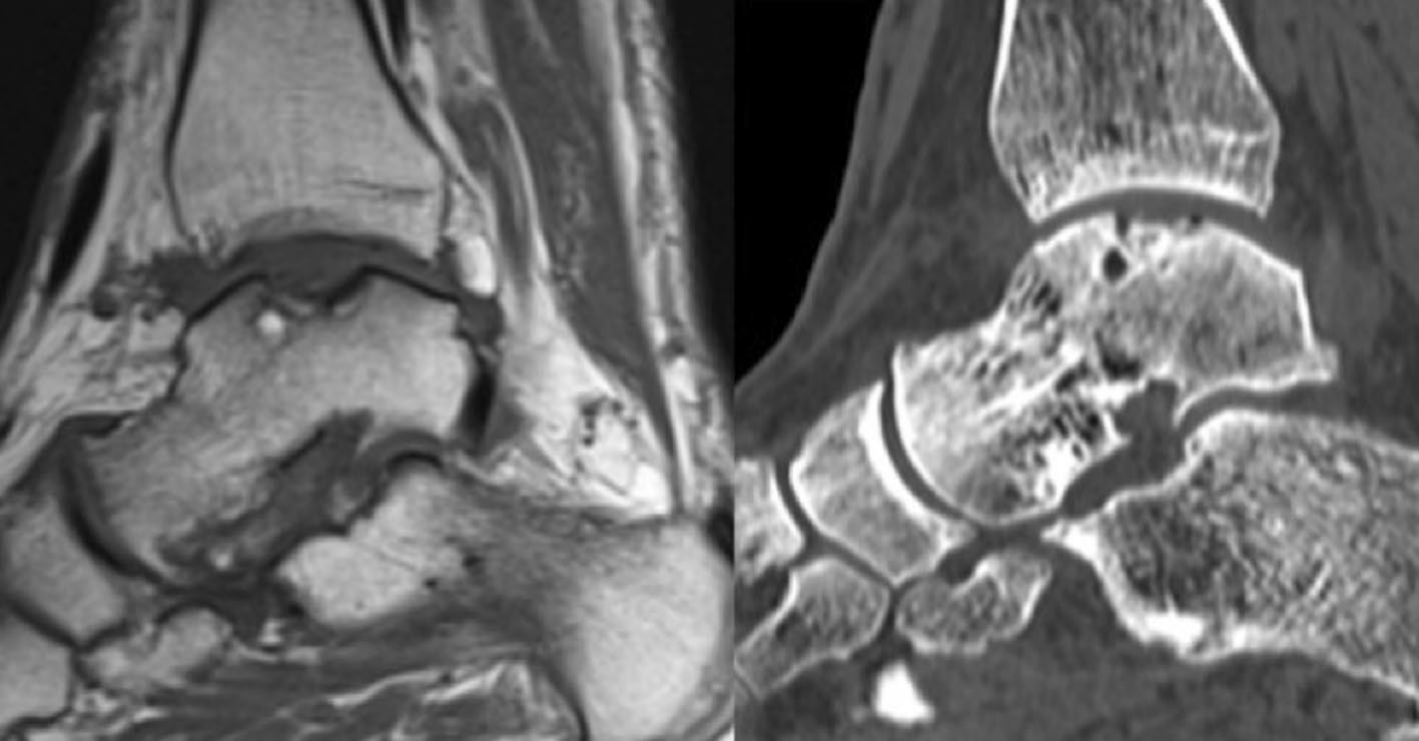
Osteochondral lesion on the talar dome: MRI compared with CT image. Sagittal view
